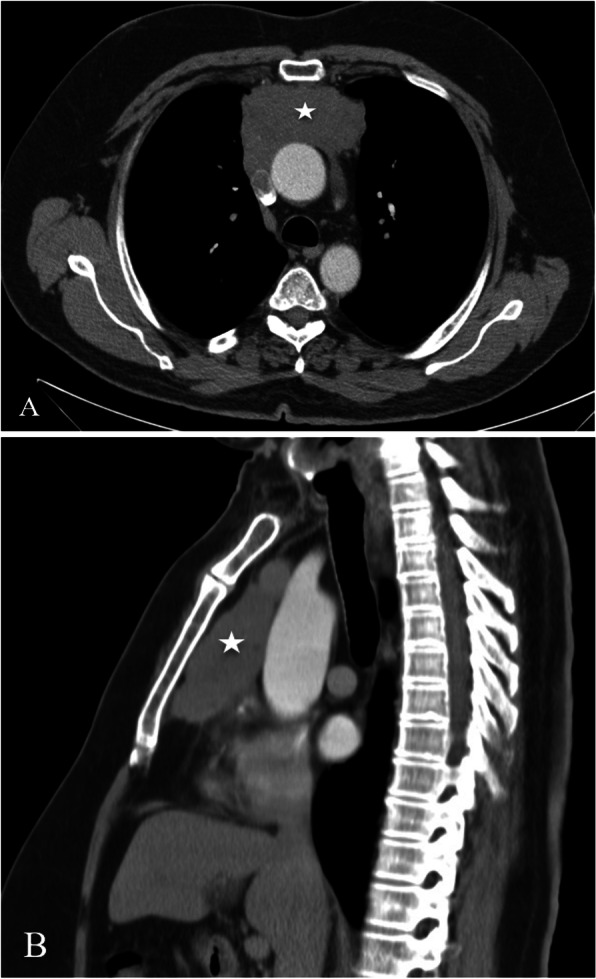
Chest CT scan with contrast, coronal view, mediastinal window showing a lobulated anterior mediastinal soft tissue mass lesion measures 10 X 6 cm (white asterisk) at the level of the aortic arch. The lesion is causing mild compression upon the SVC. However, the SVC is patent. The lesion is inseparable from the anterior wall of the SVC, shows wide area of contact with the ascending aorta, upper pericardium and associated with mild pericardial effusion
